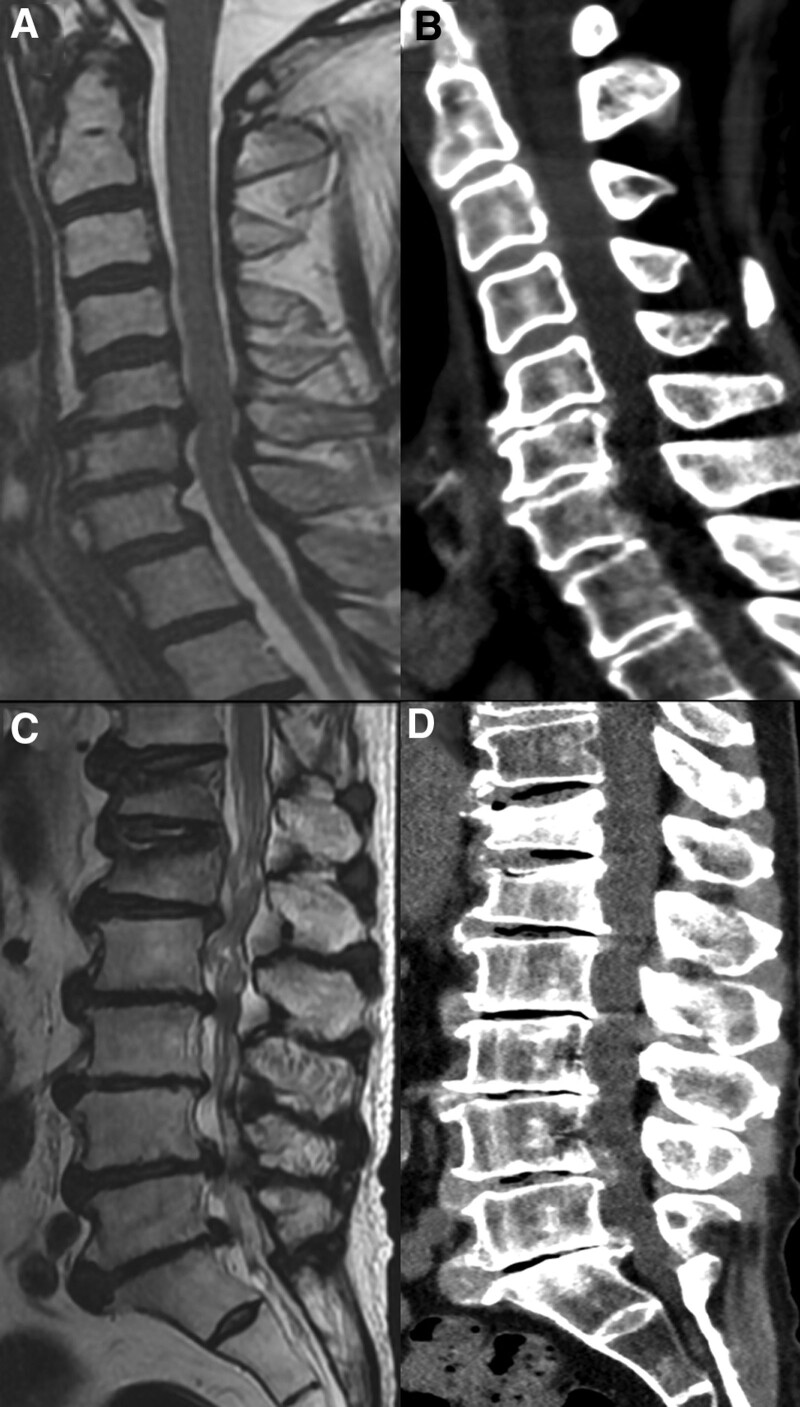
Imaging examination of cervical and lumbar vertebras. (A) MRI of cervical in sagittal view. (B) CT of cervical in sagittal view. (C) MRI of lumbar in sagittal view. (D) CT of cervical in sagittal view. CT = computerized tomography scan, MRI = magnetic resonance imaging.
